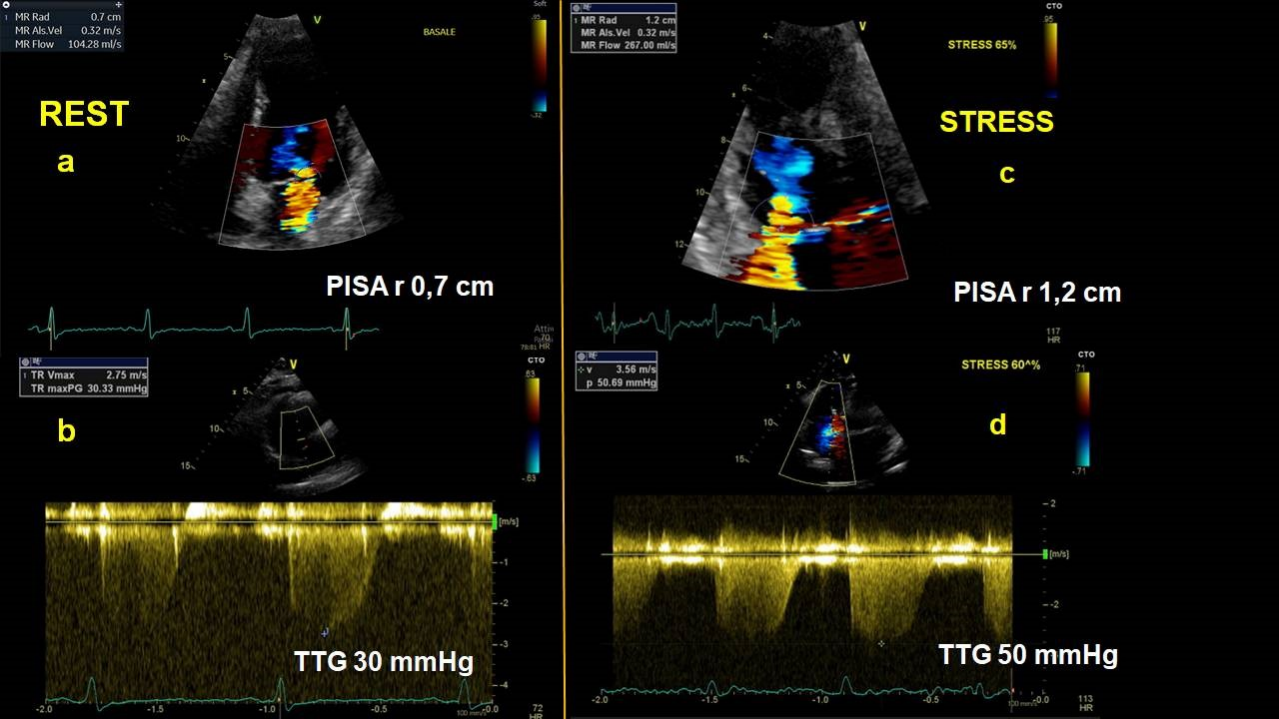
Mitral regurgitation. Stress echocardiography in a patient
